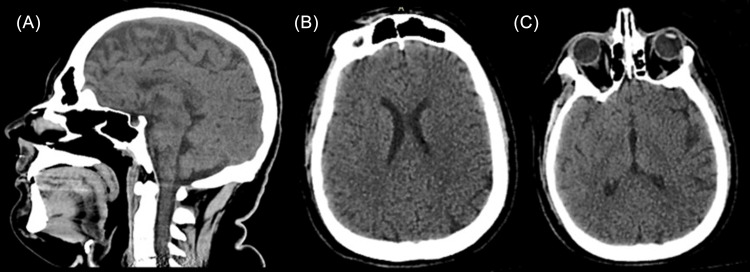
Brain computed tomography (CT) scan with no evident pathologies. (A) Sagittal view of the brain with visible structures and normal appearance. (B,C) Axial views of the brain tissue appears symmetrical without any signs of masses, hemorrhage, or edema.
